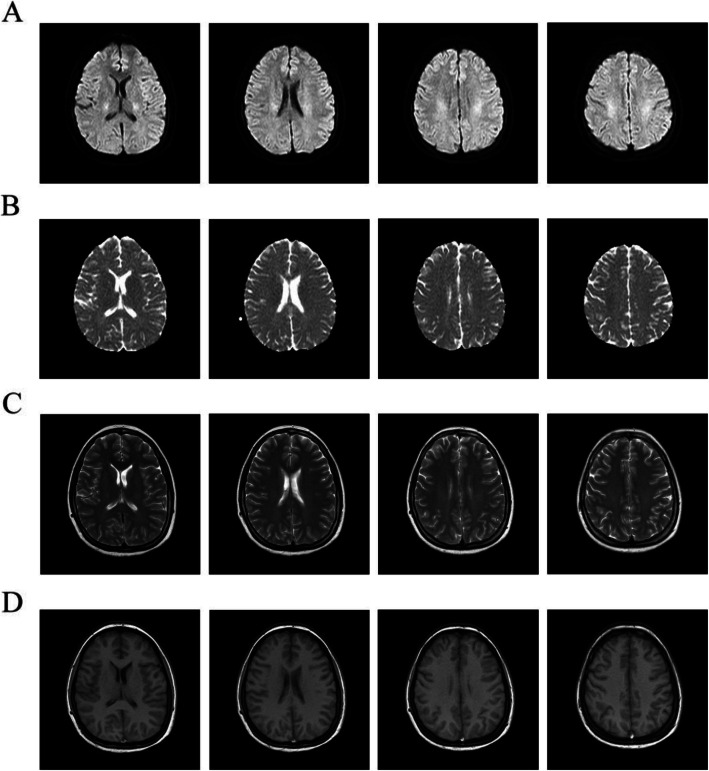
The post-intervention brain MRI. The DWI (a) and ADC (b) images showed minimal abnormal signal at the corpus callosum and radiate. No abnormality was found in T2-weighted axial images (c) and T1-weighted images (d)
